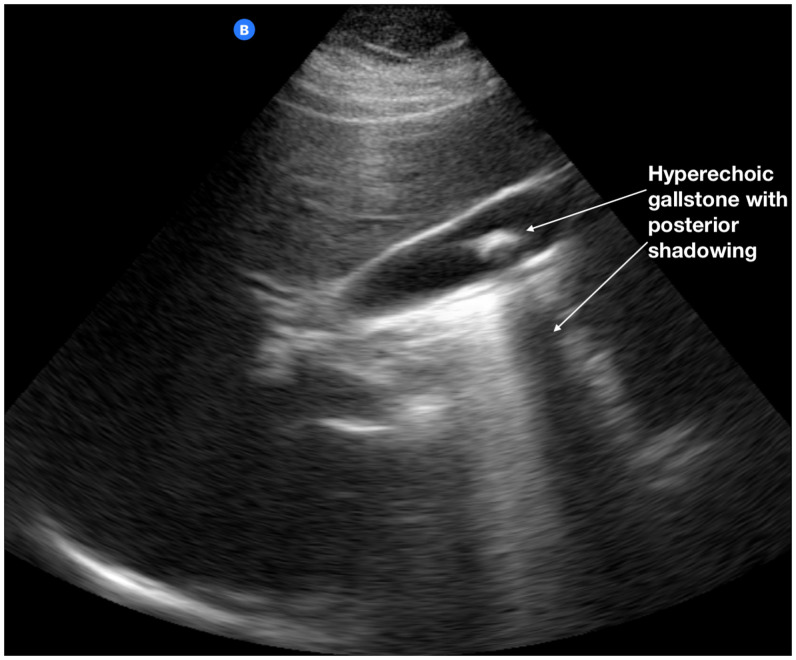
A single, large gallstone identified using the Butterfly iQ ultrasound probe in the course of screening performed by nonexpert POCUS operators. This POCUS image demonstrates the classical ultrasound characteristics of gallstones including a highly reflective echo from the anterior surface of the gallstone and marked posterior acoustic shadowing. With change of the subject’s position, the gallstone also typically changes position, which is readily detected during the POCUS examination. POCUS = point-of-care ultrasound.
